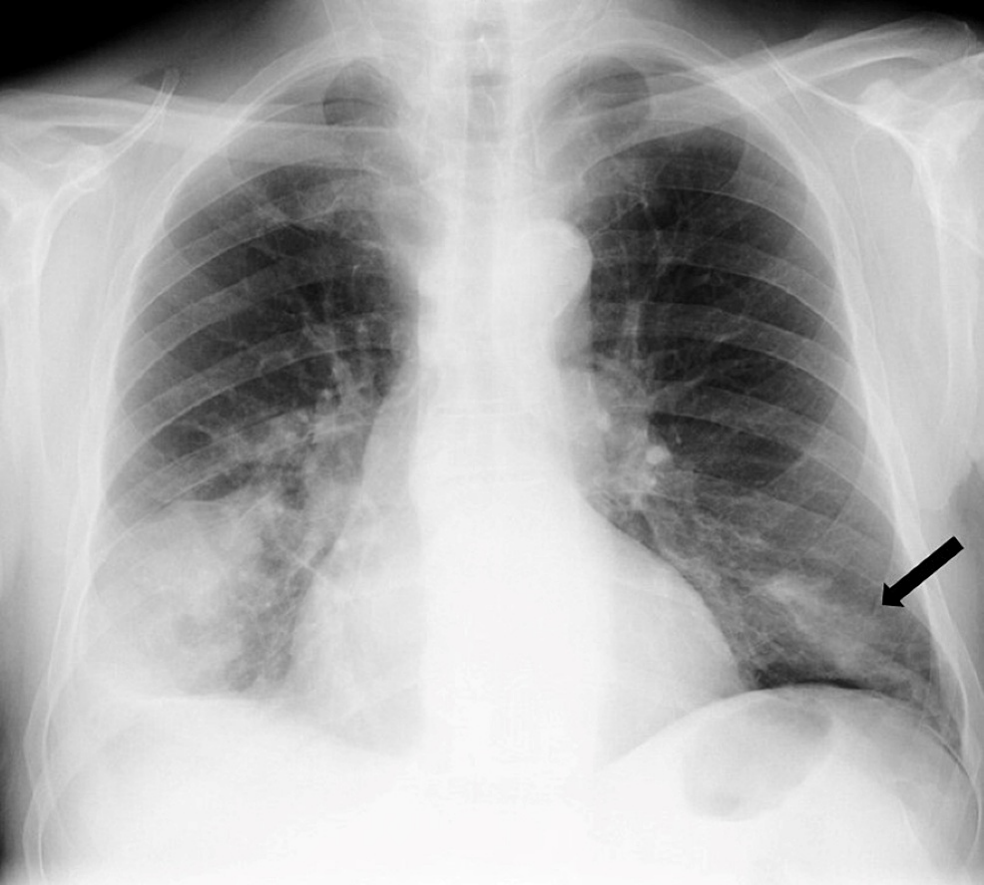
Chest X-ray obtained one week after the first observation in the emergency department, with a new condensation in the left inferior pulmonary lobe (black arrow).
